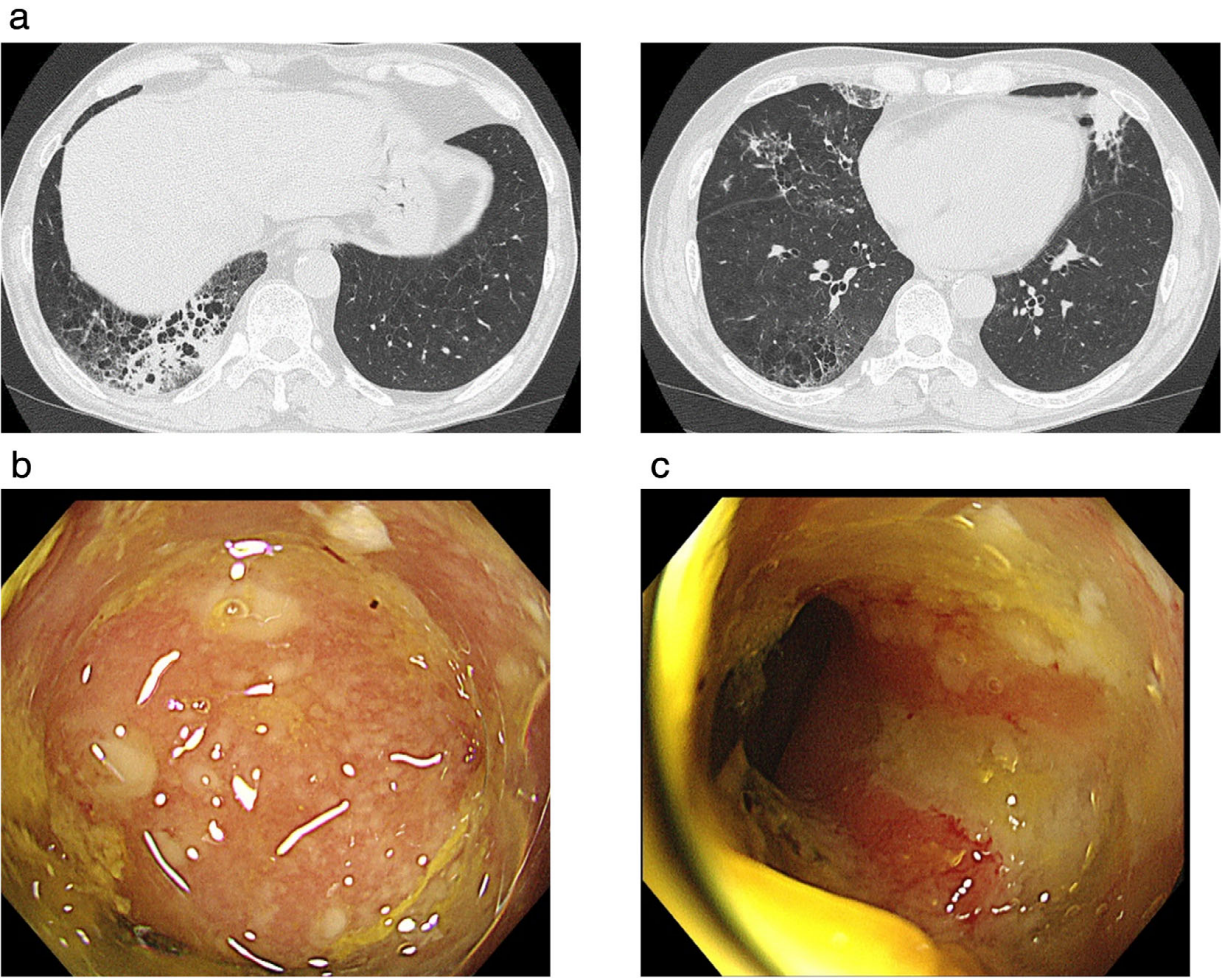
Image of immune‐related adverse events (iRAEs). (a) Chest computed tomography (CT) scans showing pneumonitis in the right lower lobe (left); the right middle and lower lobes, and the left lingular segment (right). (b and c) Colonoscopy revealed multiple ulcerations in the descending colon
